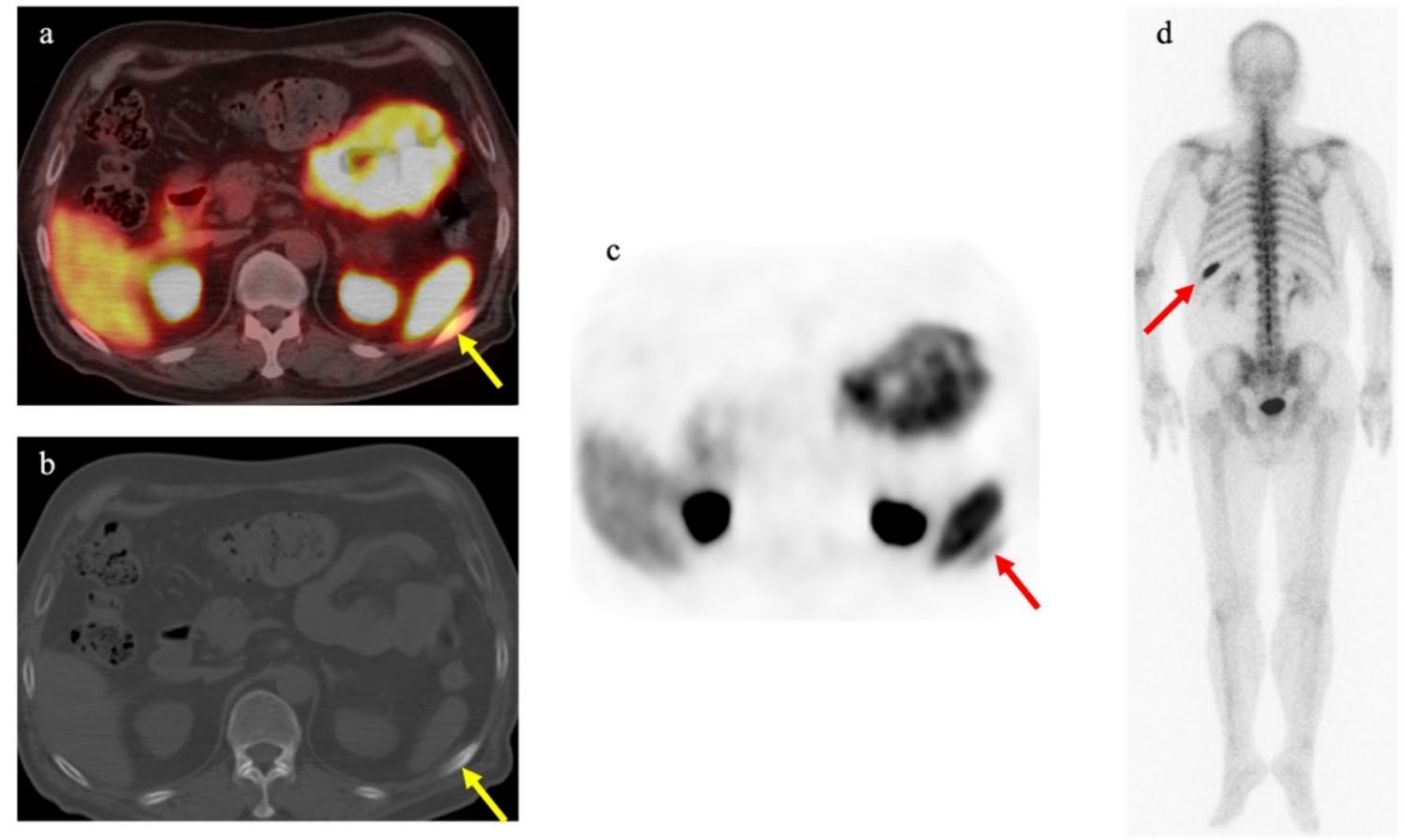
PET/CT using ^68^ Ga-PSMA-11 in a 69-year-old man with biochemical recurrence following intensity-modulated radiation therapy (cT3bN0M0, Gleason score 5 + 4). PSA level increased to 3.10 ng/ml. Focal uptake was observed in the left dorsal rib, but identification was challenging due to intense physiological uptake by the spleen. CT revealed slight sclerosis at the rib lesion site. Bone scintigraphy showed high uptake along the longitudinal axis, a typical pattern for bone metastasis. a Transaxial PET/CT fusion image. b Transaxial CT. c Transaxial PET. d Posterior view of whole-body bone scintigraphy
